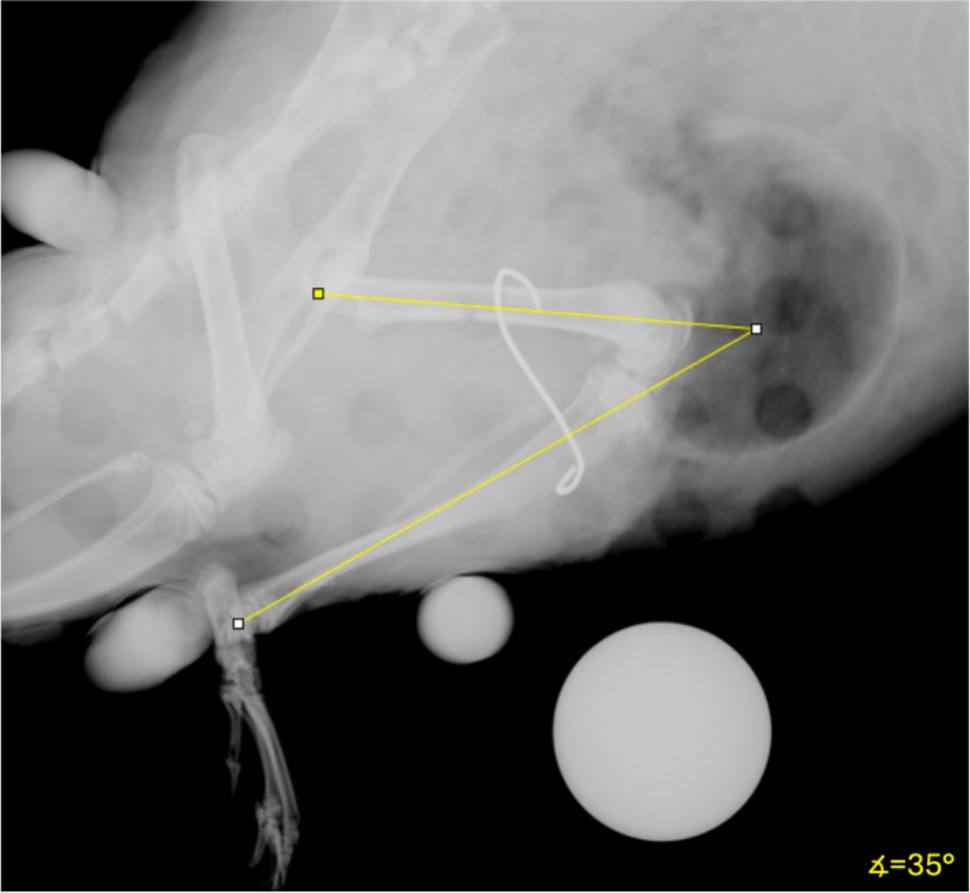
Lateral X-ray of the knee joint. A joint angle of 35° was fixed using a K-wire arthrodesis
